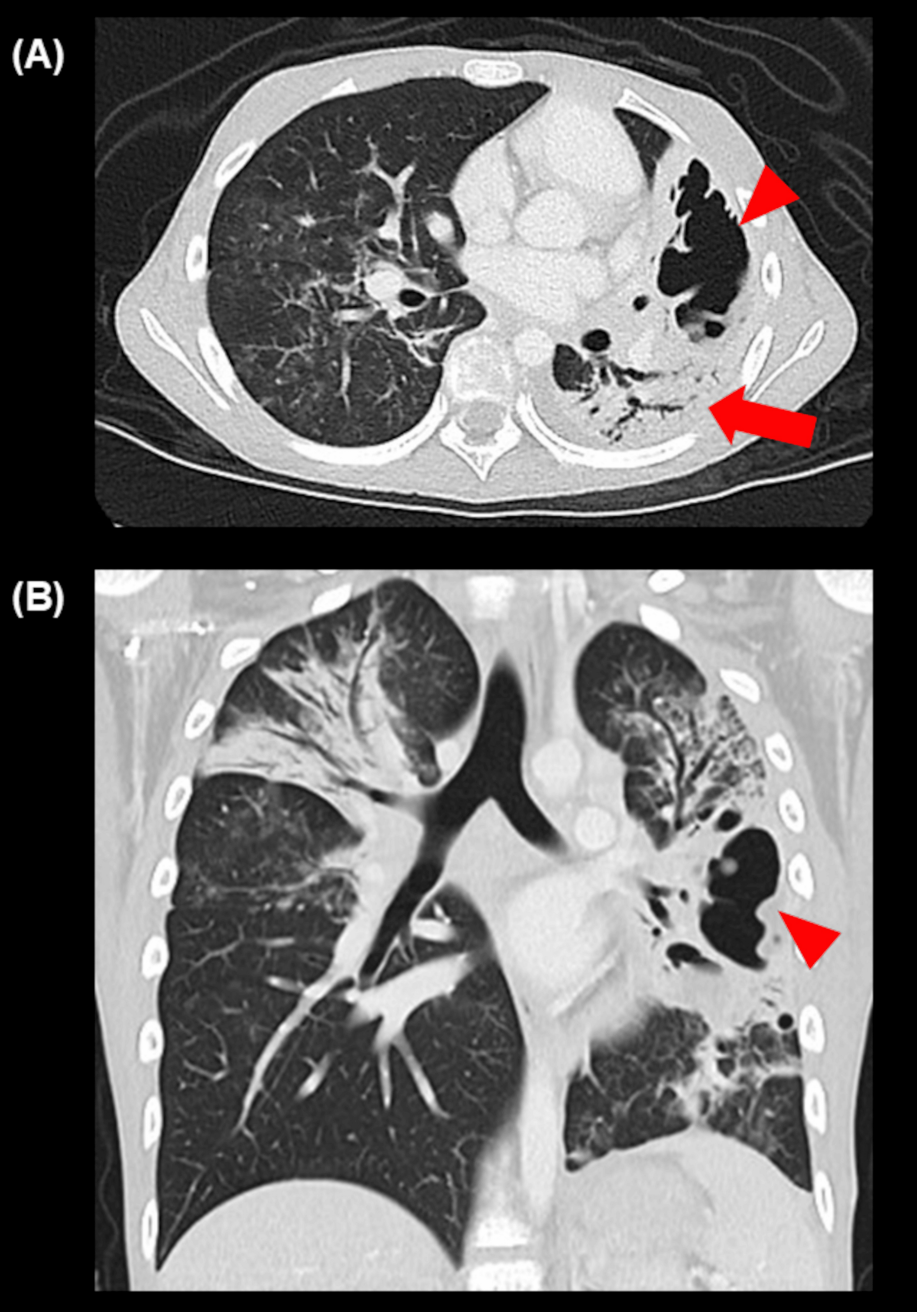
Computed tomography scan one week after discharge from the pediatric intensive care unit. Computed tomography scan showed residual consolidation (A, arrow) and cavitation (A, B, arrowhead).
